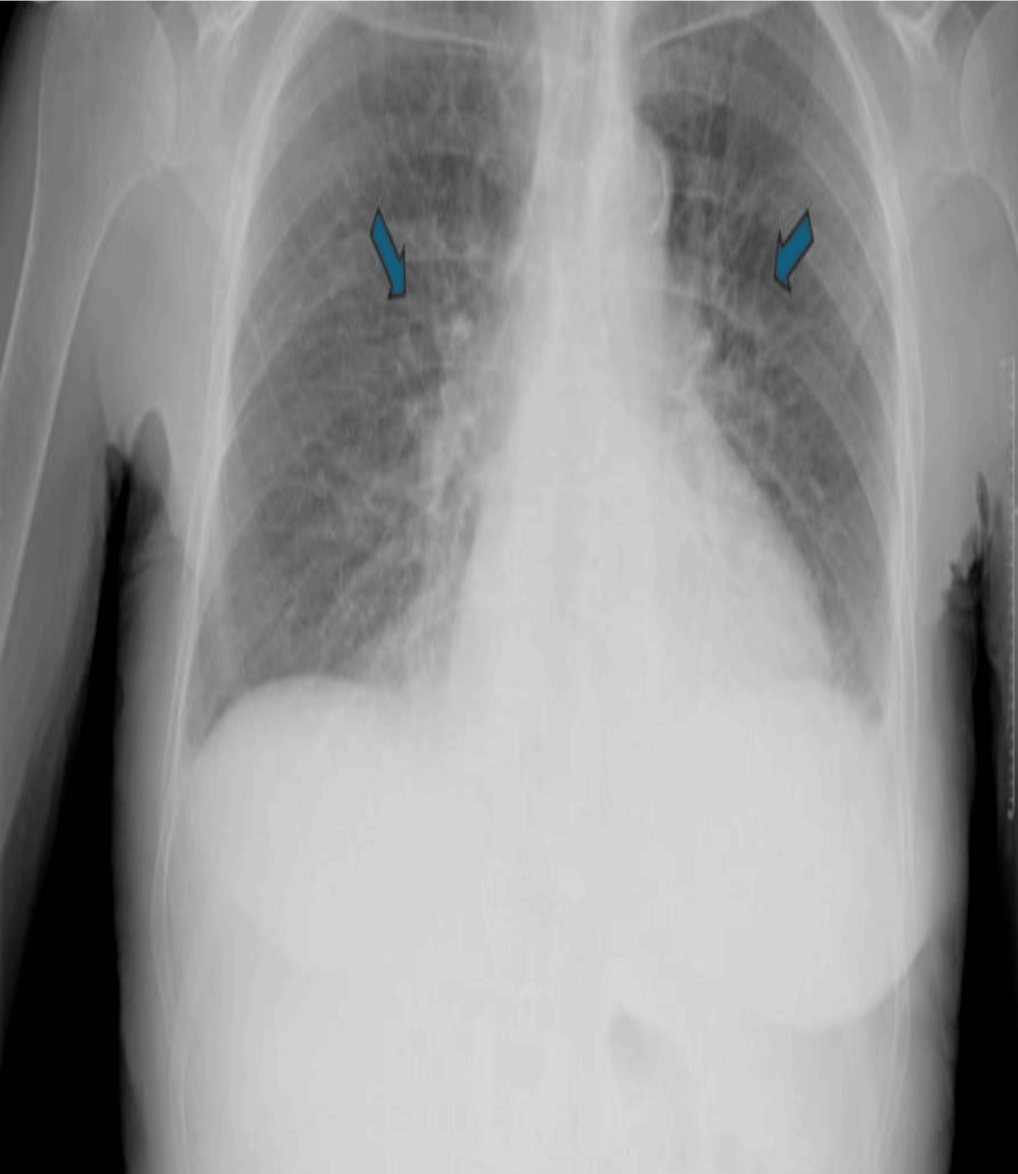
Chest X-ray during hospital stay The arrows indicate pulmonary congestion.
